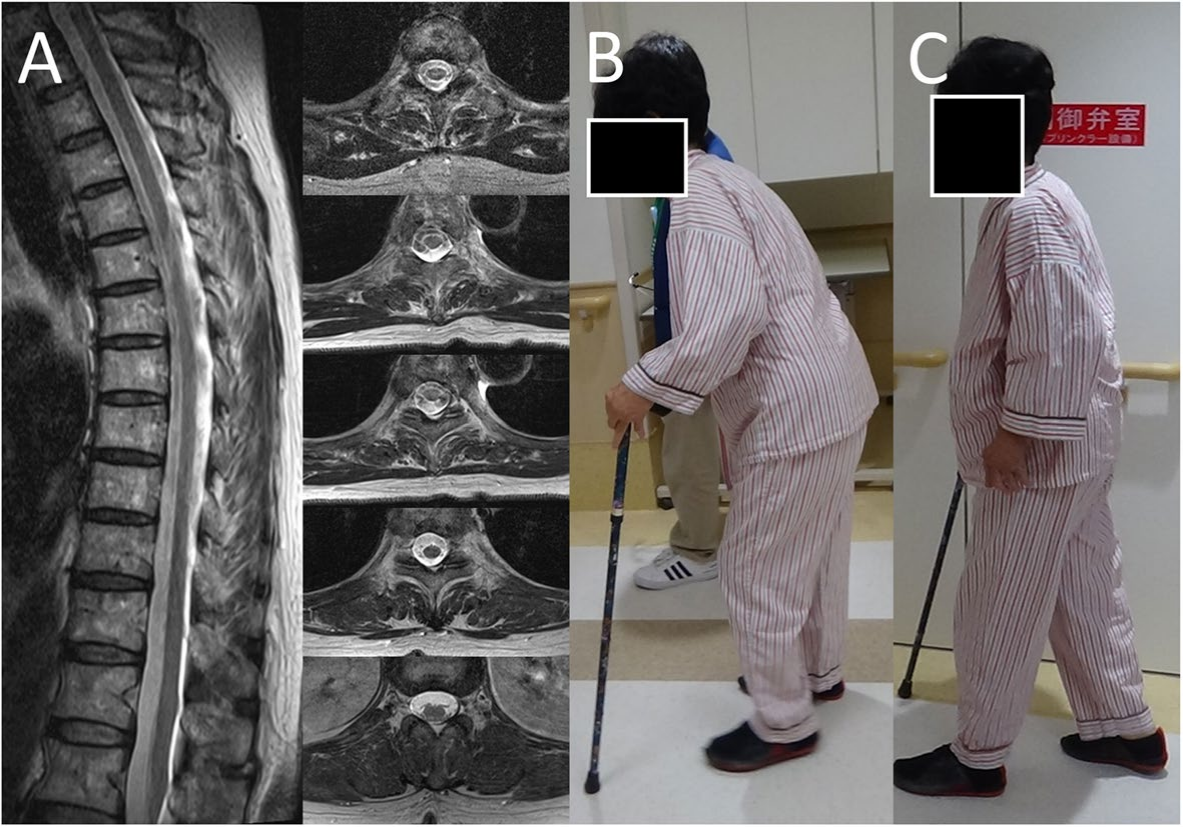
Change in patient’s walking posture before and after steroid treatment. A The MRI T2-weighted imaging (T2WI) revealed slight paraspinal muscle atrophy with no spinal kyphosis. Images of the patient before (B) and after (C) high-dose intravenous methylprednisolone therapy for 3 days, resulting in marked improvement of walking
